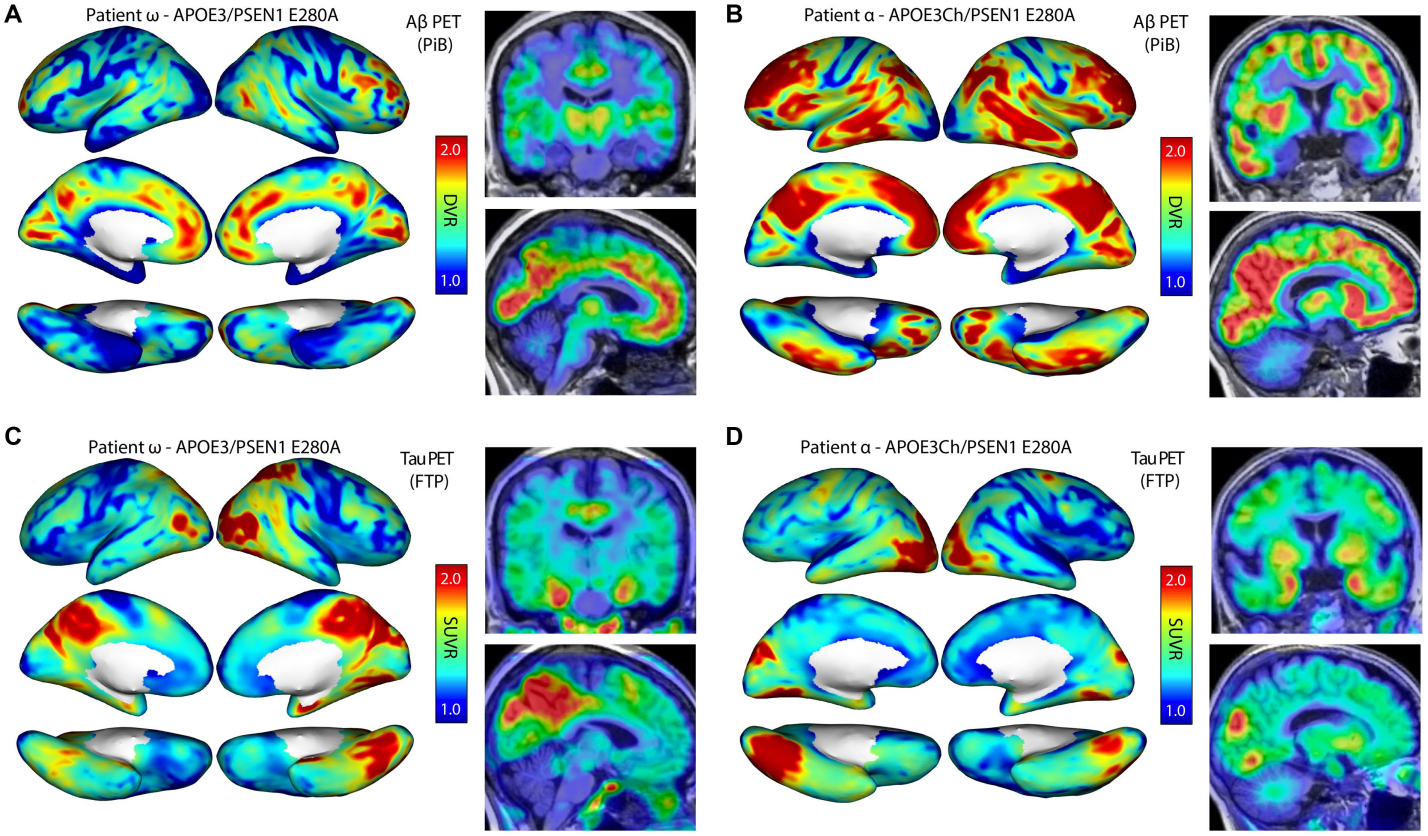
Autosomal dominant Alzheimer’s disease (ADAD) patient *in vivo* neuroimaging. Patient ω is a non-protected, Paisa kindred control patient and was scanned for amyloid β (A) and tau burden (C). Patient α is an AD-protected Paisa kindred patient and was previously described and scanned for amyloid β (B) and tau burden (D) (Arboleda-Velasquez et al., 2019). Scans indicated elevated amyloid β burden in patient α (B) compared to patient ω (A). Tau burden was elevated in patient ω (C) over patient α (D), with a marked increase in medial temporal and parietal regions. PiB, Pittsburgh Compound B; FTP, Flortaucipir; DVR, Distribution volume ratio; and SUVR, Standardized uptake value ratio.
